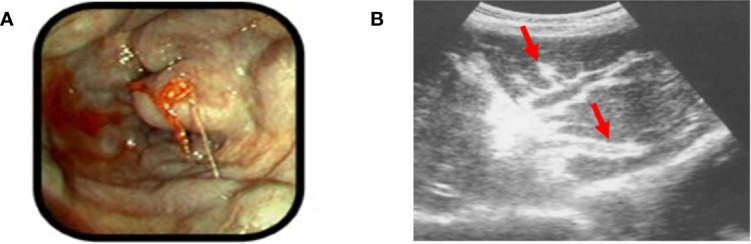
Examples of HSS (A). An example of bleeding esophageal varices: note the bulging esophageal mucosa indicative of underlying varicies, with signs of active bleeding (Picture taken by ES in the endoscopy unit, University of Zambia Teaching Hospital, Lusaka, Zambia.). (B) Periportal fibrosis detected by ultrasound (red arrows). Reproduced from reference (39), with addition of the red arrows by the present authors to clarify the location of the pathology.
